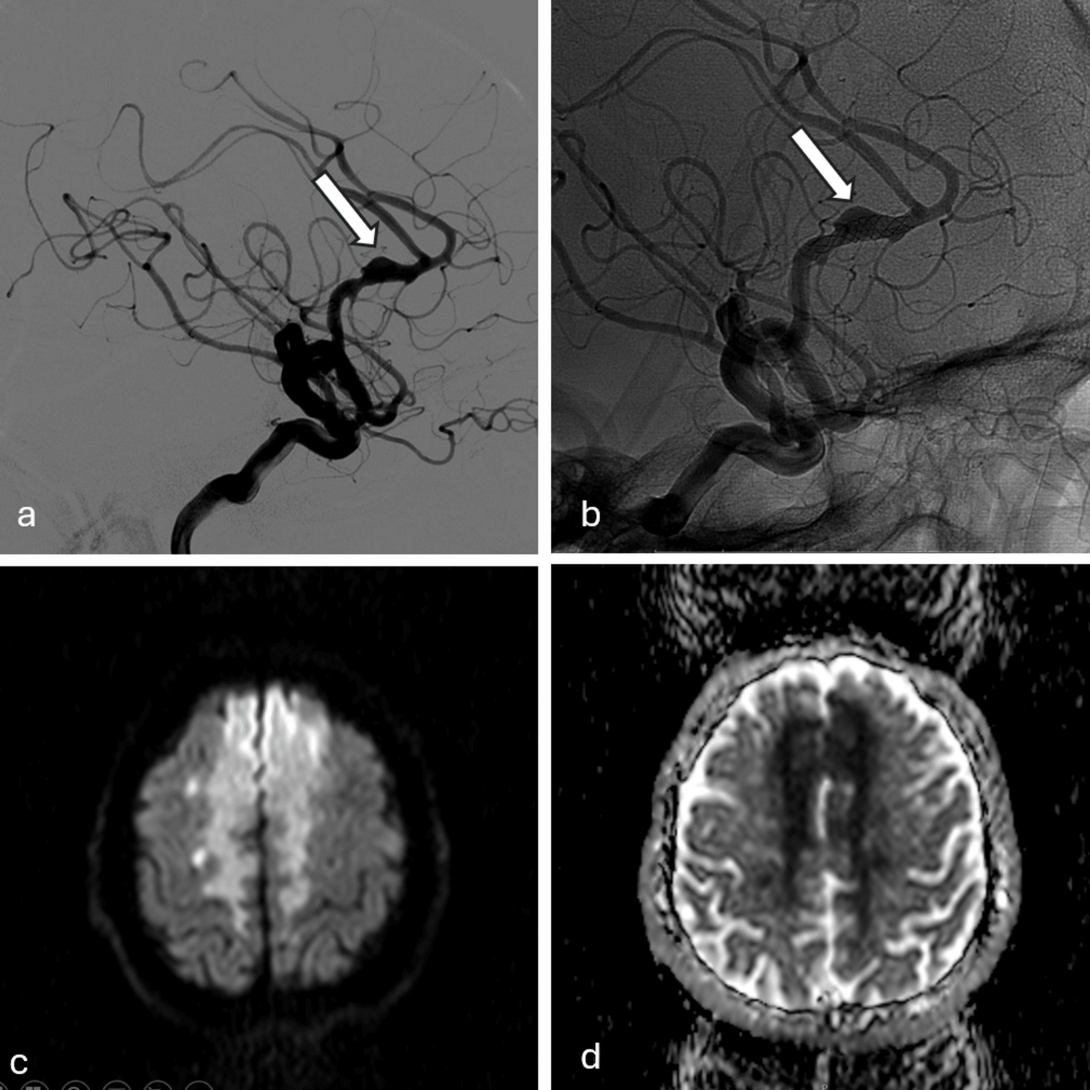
a: Fusiform aneurysm (white arrow) in the pericallosal artery in the subtracted angiography images. b: Silk stent and fusiform aneurysm (white arrow) in unsubstracted angiography images. c and d: In the diffusion-weighted imaging (DWI) trace and apparent diffusion coefficient (ADC) images an acute infarction in the anterior cerebral artery (ACA) territory.
